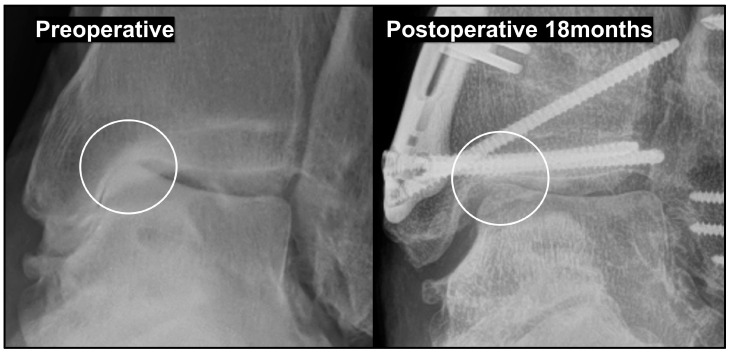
Postoperative gap formation between the medial talar dome and the plafond. The formation of a radiographic gap between the medial talar dome and the plafond is presumed to be directly associated with postoperative symptom improvement by eliminating subchondral bone contact.
